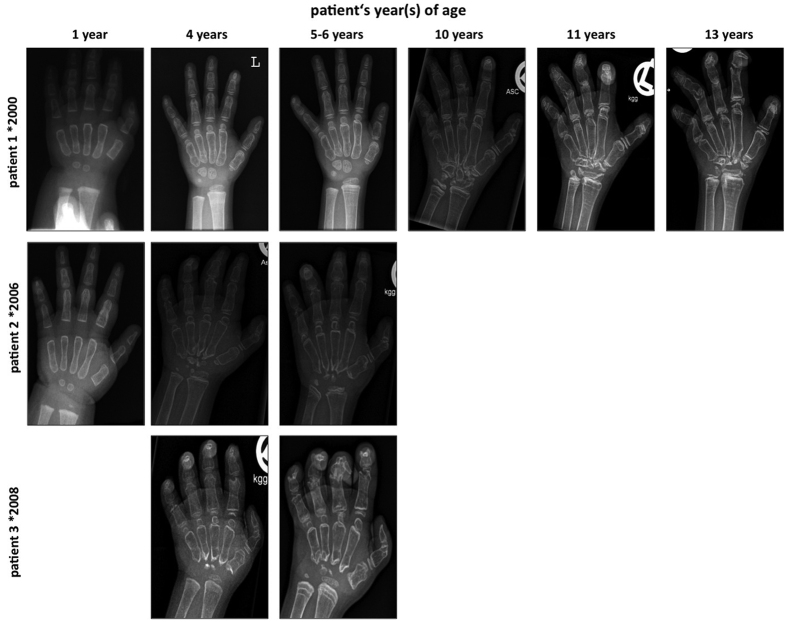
Dorsopalmar radiographs of the left hand demonstrate severe general osteopenia, progressive destruction of carpal bones and joints, multiple osteolyses, widening of phalanges and metacarpals with thinning of cortical bone and irregular epiphyses. Pronounced sklerotic metaphyseal lines most evident at the radial and ulnar bone are due to bisphosphonate therapy.
